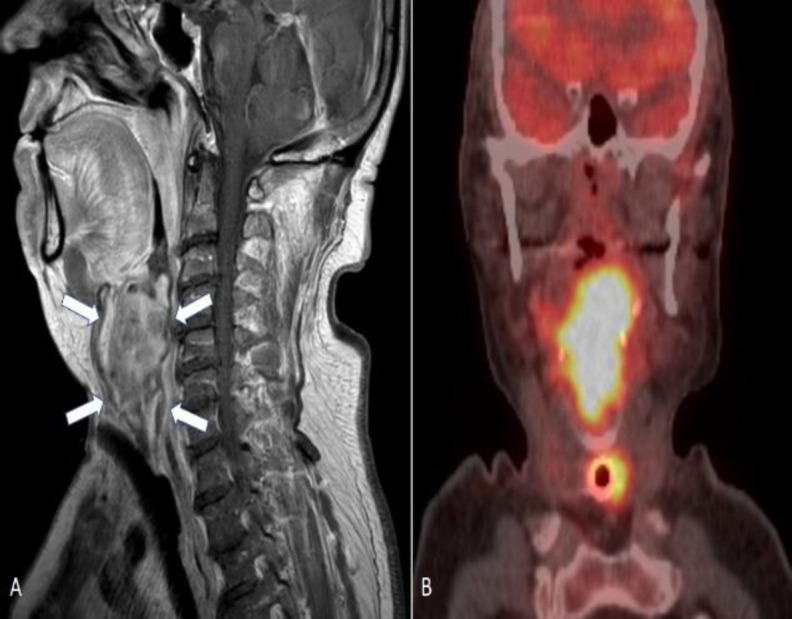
Magnetic resonance (Α) and Combined FDG-positron emission tomography (PET)/ computed tomography (CT) (B) images. (A) Sagittal MRI scan showing the mass (white arrow) that occupies oropharynx, hypopharynx, glottic and subglottic area. (B) Coronal PET/CT imaging - the tumor appears as a hypermetabolic lesion (hot spot) [Time-of-flight (TOF) reconstruction]
